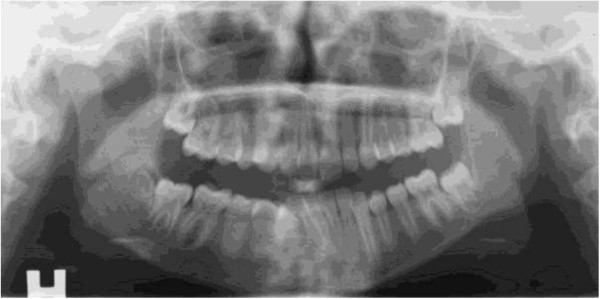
Panoramic radiograph on a patient with JIA with example of common alteration, flattening, on the left TMJ. Right side (H) is normal.
